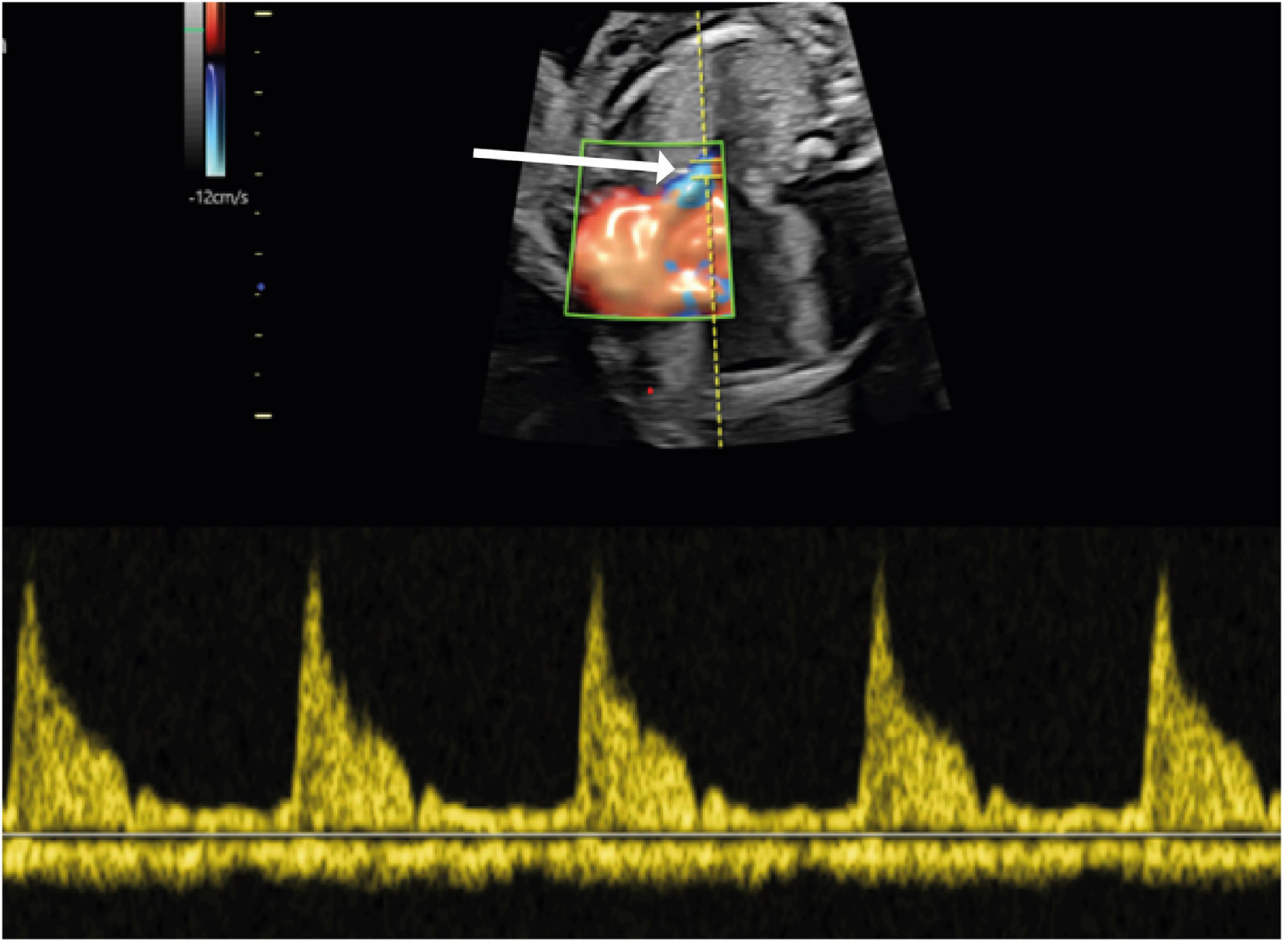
Two-dimensional fetal echocardiogram with color flow and pulsed-wave Doppler obtained at 21 weeks of gestation, transverse plane, demonstrates the spectral Doppler display from one presumed pulmonary vein (*arrow*) with an unclear site of drainage; the spectral Doppler profile is abnormal, as the pattern below the baseline does not demonstrate conventional phasic flow patterns seen with pulmonary veins.
